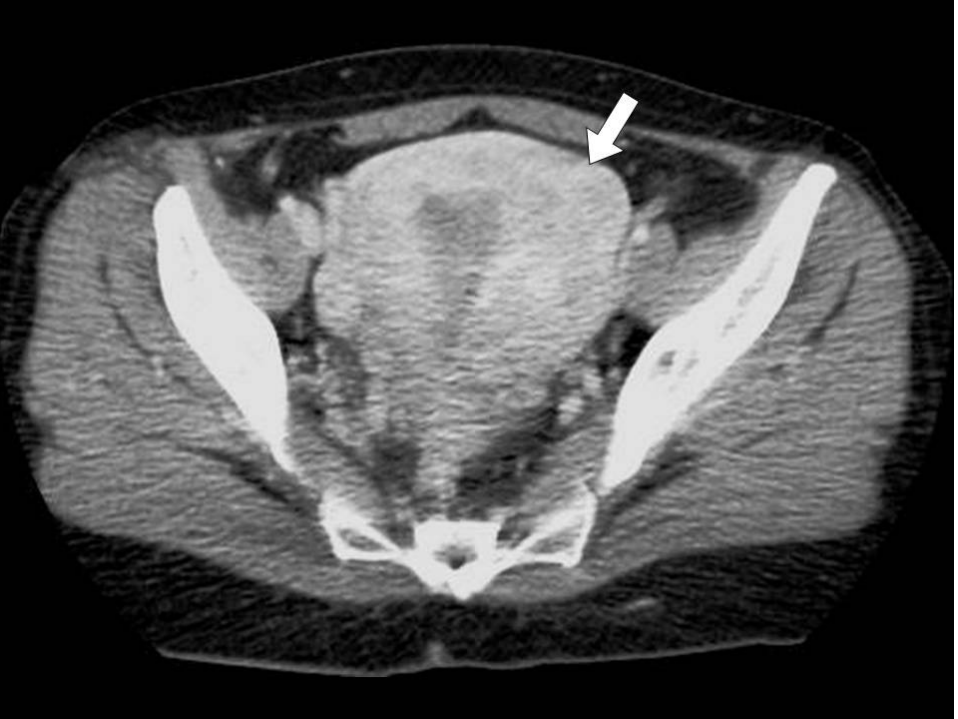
Contrast-enhanced axial computed tomography scan of the pelvis. Contrast-enhanced axial computed tomography scan of the pelvis demonstrates an enlarged uterus with deformed contour (arrow), consistent with leiomyomatous uterus. The uterus enhances heterogeneously and vividly in a similar way compared to the previously described mass in the left lower quadrant (figure 3B).
